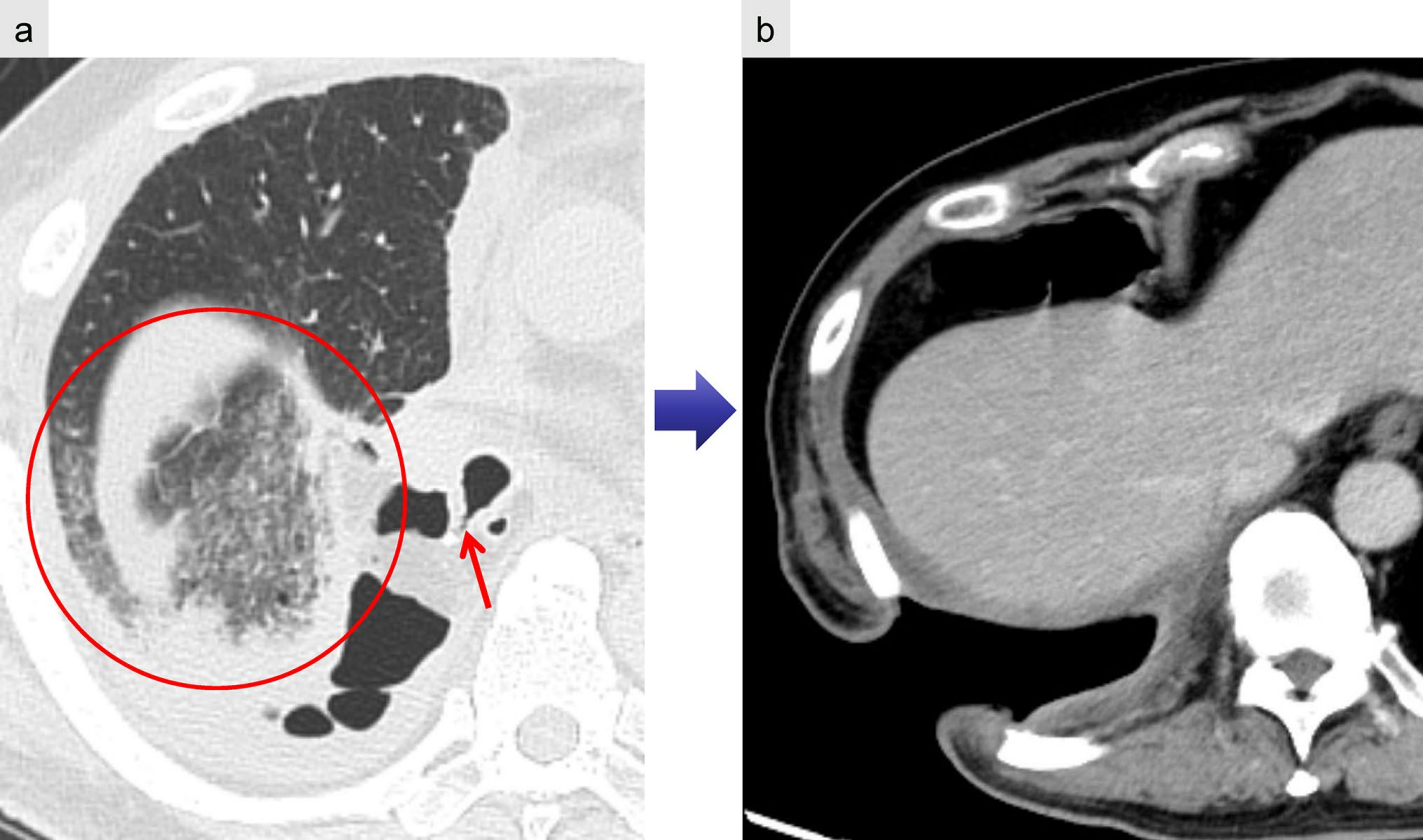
Bronchopleural fistula (BPF). Following right lower lobectomy (a, b), BPF with aspiration pneumonia and empyema are observed. CT (a) demonstrates a bronchial stump fistula (arrow) with adjacent air and fluid accumulation within the pleural cavity. The lung parenchyma shows ground-glass opacities (circle), consistent with aspiration pneumonia. An open-window thoracostomy was performed to manage the empyema (b)
